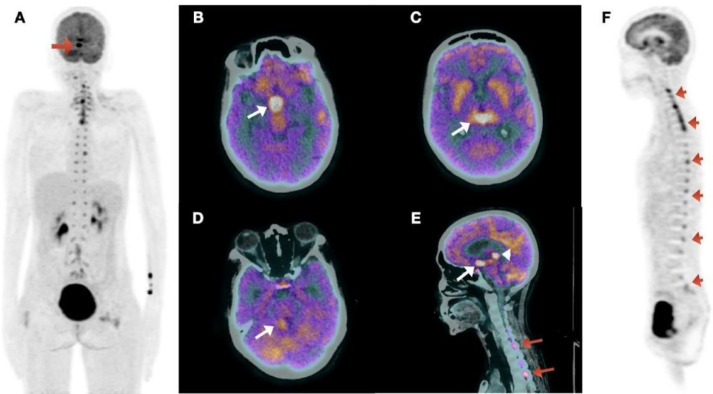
18F-FDG PET/CT: Increased tracer uptake in intracranial and paravertebral regions (A: MIP image). On fused axial images there is increased tracer uptake in suprasellar region (B) and pineal gland region (C) and cerebellar vermis region (D). Sagittal fused image (E) reveals increased tracer uptake in pineal gland (arrow head) and suprasellar regions (arrow), and cervical nerve roots (red arrow). On sagittal PET image there is increased tracer uptake in cervical spinal cord, lumbar, and sacral nerve roots (F)
